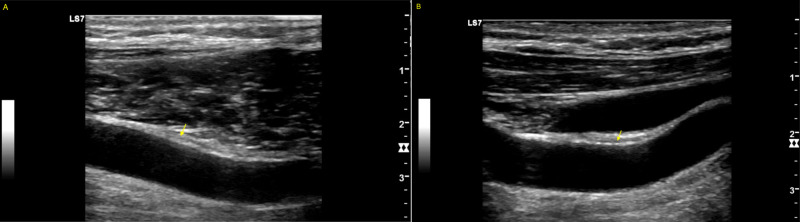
Carotid Artery Ultrasound for a Patient with Mild COVID-19 Carotid ultrasound showed no significant atheroma in the right carotid artery (A) and left carotid artery (B).
